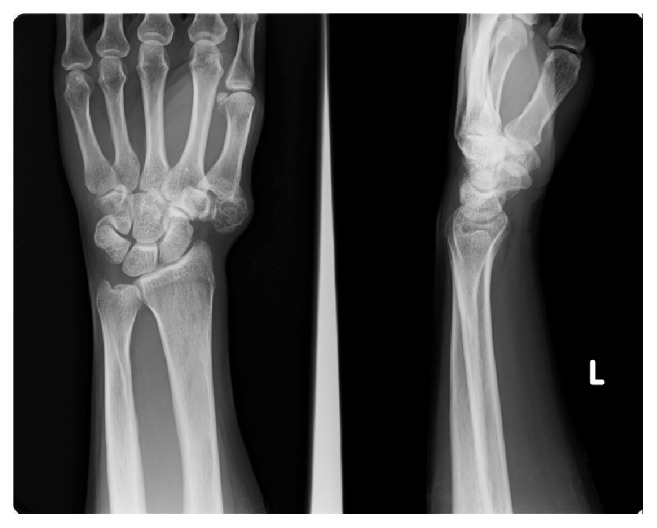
PA and lateral plain X-ray of the left hand showing a soft tissue lesion with calcifications adjacent to trapezium and the base of the first metacarpal bone.
